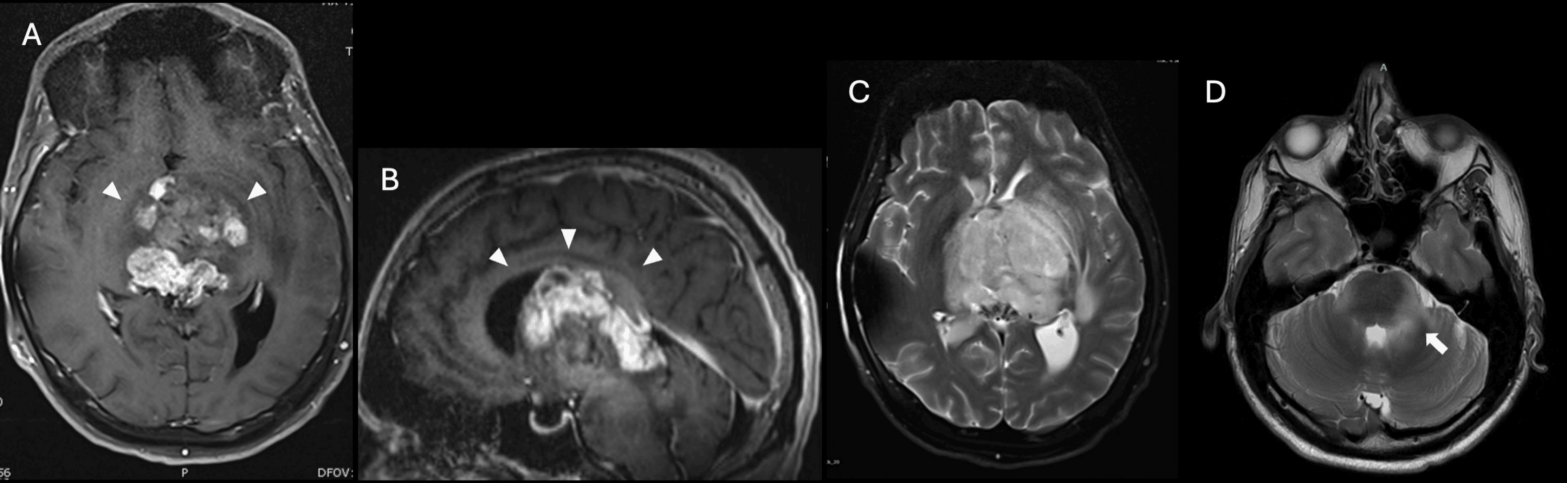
Tumor progression on MRI Follow-up MRI performed 11 months after diagnosis with interval increase in mass size to 4.6 × 5.1 × 5.2 cm. (A) Axial and (B) sagittal T1W enhanced images show an increase in tumor size throughout the third ventricle and increased regions of enhancement, with extension into the basal ganglia, tectum, and corpus callosum (arrowheads). (C and D) Axial T2W images show heterogeneous T2 signal with severe local mass effect and invasion into the bilateral thalami, left basal ganglia, and new extension into the pons and left middle cerebellar peduncle (arrow). MRI: magnetic resonance imaging
